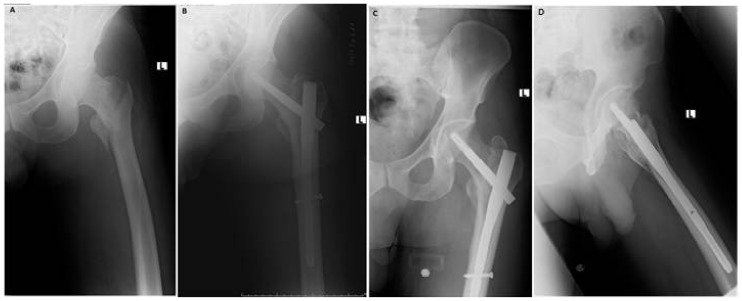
Type A2 fracture (A). Immediate postoperative AP radiograph showing a neck-shaft angle of 130° (B). The 6 months follow up AP radiograph showing a neck-shaft angle of 130° and union of the fracture (C). Lateral view at 6 months follow up (D).
